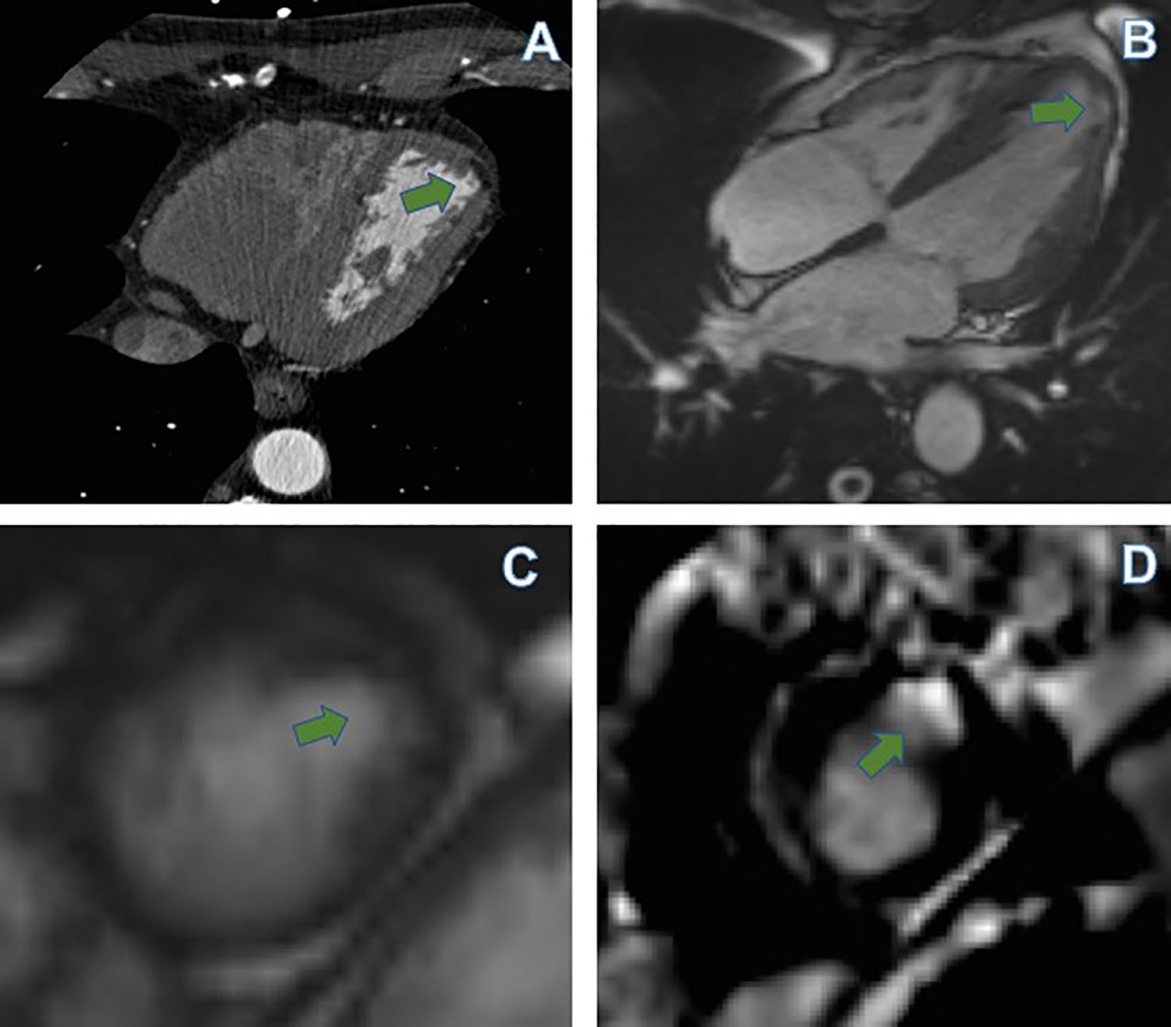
(A) Enhanced cardiac CT axial view shows almost similar but smaller focal aneurysm in the lateral apical segment, (B, C) cardiac MR 4-chamber and short axis views confirm the presence of aneurysm (arrow), and (D) cardiac MR delayed enhancement phase shows abnormal subendocardial enhancement along the same area in the lateral apical segment.
